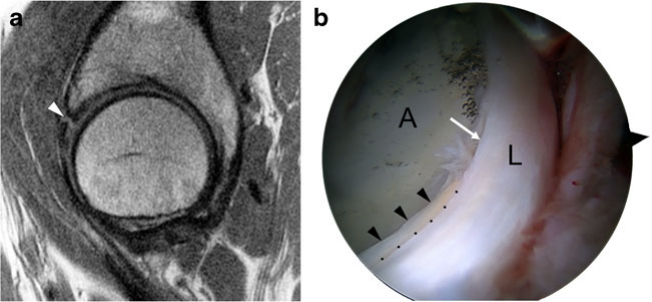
A 46-year-old woman with combined cam and pincer impingement. a Sagittal PD-weighted MRI demonstrates separation at the chondrolabral junction (*black arrow*). b Arthroscopic photo in the same patient demonstrates acetabular cartilage (A), labrum (L), and the transition zone between (*dots*). There is chondrolabral separation between 1 and 3 o'clock (*white arrow*) and chondral delamination (*black arrowheads*) adjacent to the transition zone
